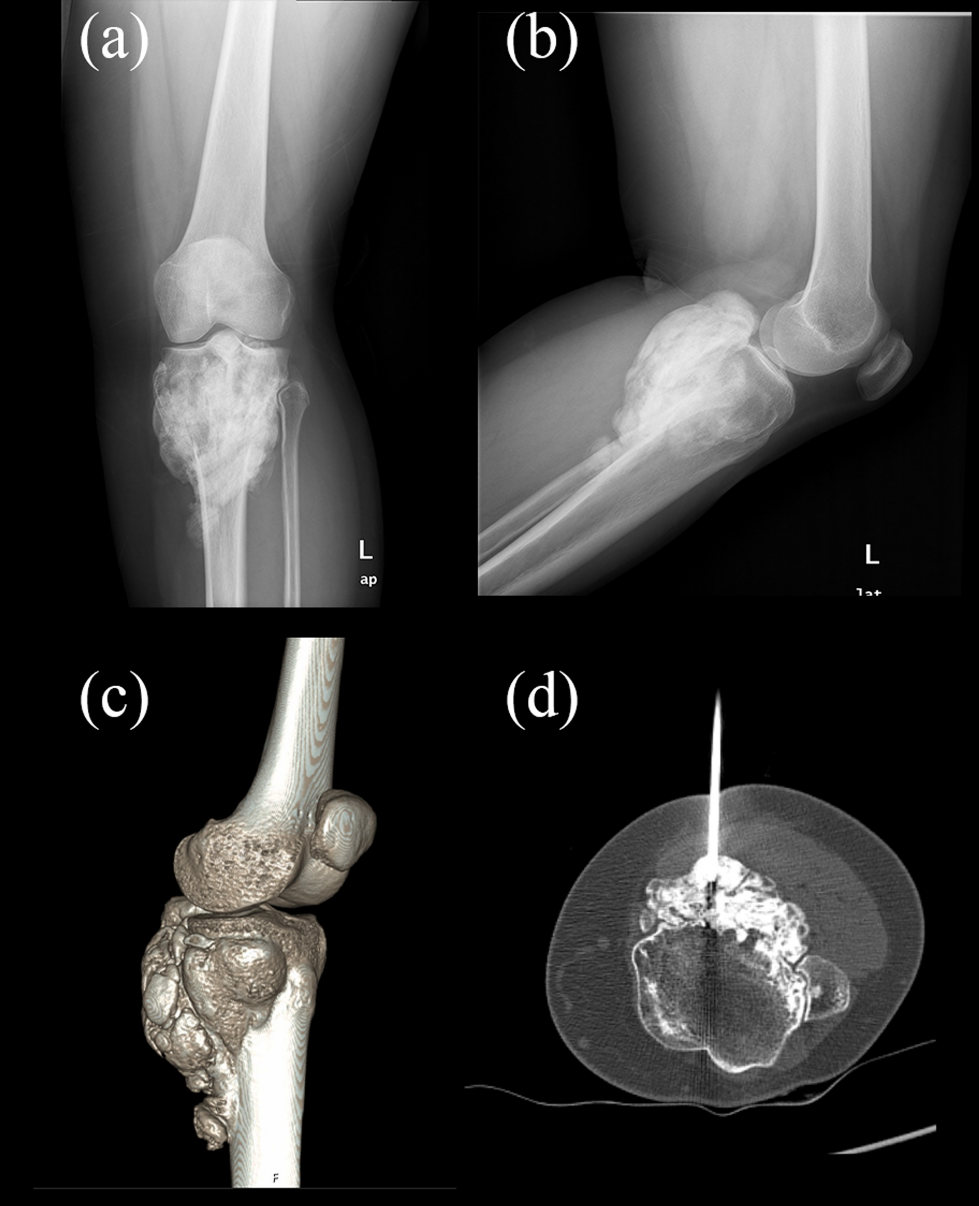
Preoperative imaging of the patient (a-b) Preoperative X-rays; (c) 3D CT image; (d) CT-guided percutaneous biopsy CT: computed tomography
